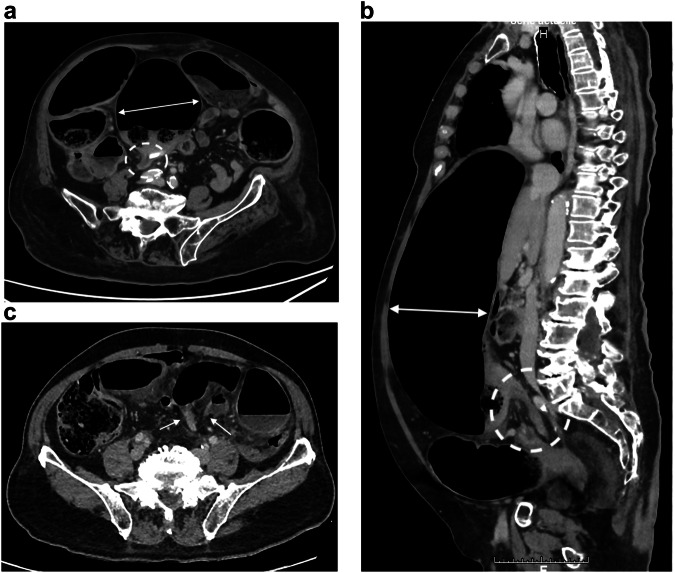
Volvulus of the sigmoid. Axial and sagittal portal phase CT (a, b) in an 86-year-old woman shows distension of the large bowel (arrows) above a unique “beak-sign” (dotted circle) corresponding to the transition point of an organo-axial sigmoid volvulus. c Volvulus of the sigmoid. Axial portal phase CT (c) in a 91-year-old man shows a mesenteric-axial sigmoid volvulus as suggested by the presence of two adjacent “beak-signs” (arrows)
